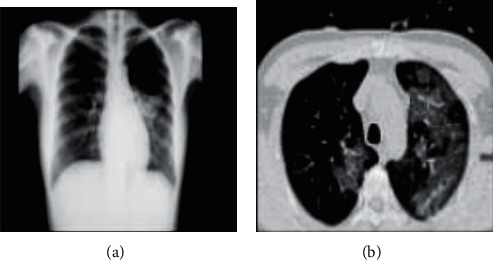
(a) CXR image; (b) CT image.
